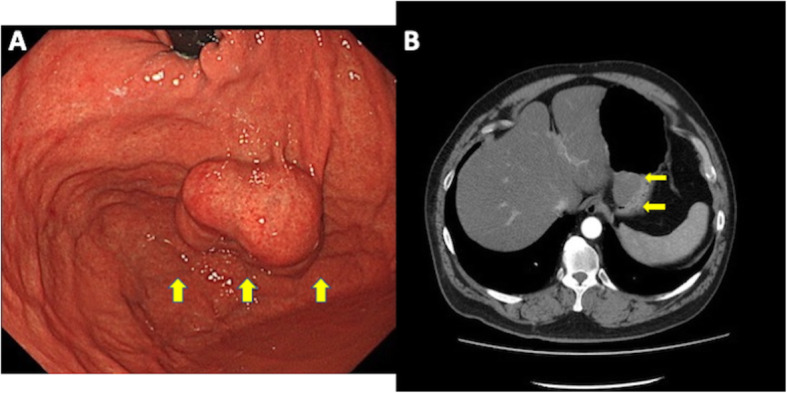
Preoperative imaging found a large SMT (yellow arrows) at the gastric fundus. a Endoscopy. b CT scan
